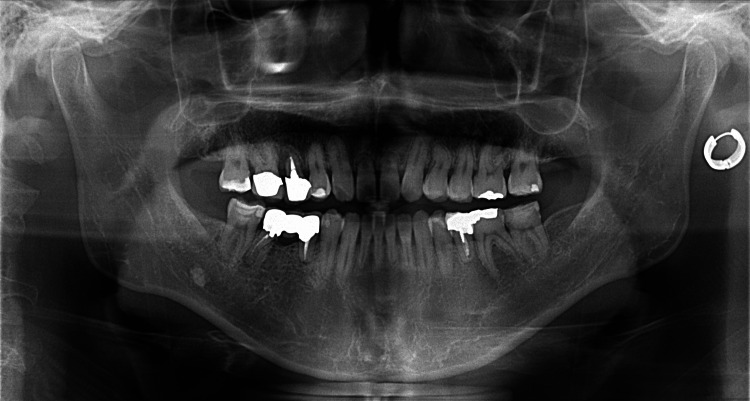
Panoramic radiograph during the treatment Panoramic radiograph after setting a fixed partial denture on the lower right second premolar and the distal root of the first molar.
